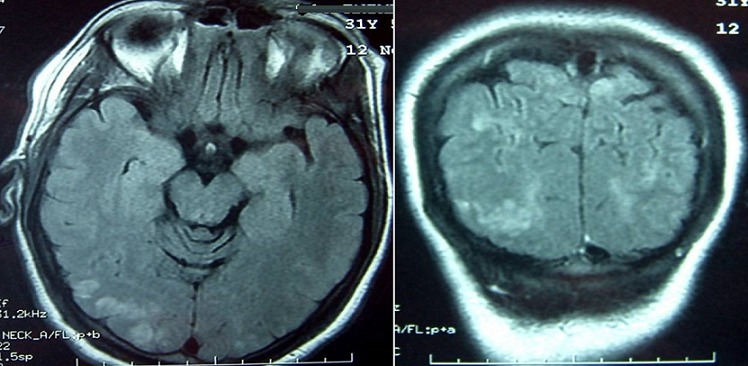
Brain MRI, hypersignal T2 and flair sequences, interesting the cerebral cortex, parietal and occipital sub-corticale
